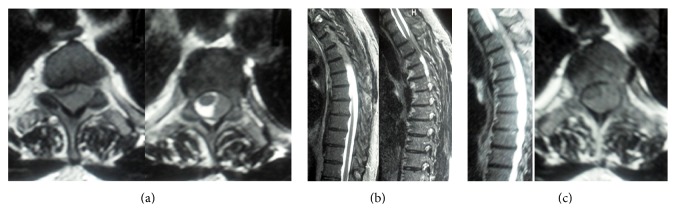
(a) Axial T2-weighted MRI shows thoracic epidural lesion with cord compression at the T2–T4 levels, extending to the paraspinal muscles. (b) Sagittal T2-weighted MRI shows dural mass. (c) Images showing T2-weighted sagittal and axial MR scans.
